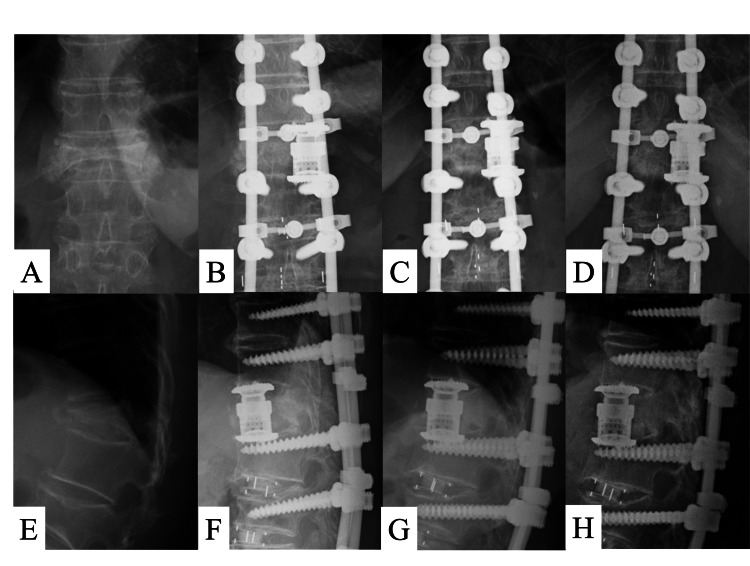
Representative case. The case of a 75-year-old woman with T12 vertebral burst fracture. A-D) Preoperative, postoperative, 1-year follow-up, and 3-year follow-up anterior-posterior radiographs. E-H) Preoperative, postoperative, 1-year follow-up, and 3-year follow-up lateral radiographs.
